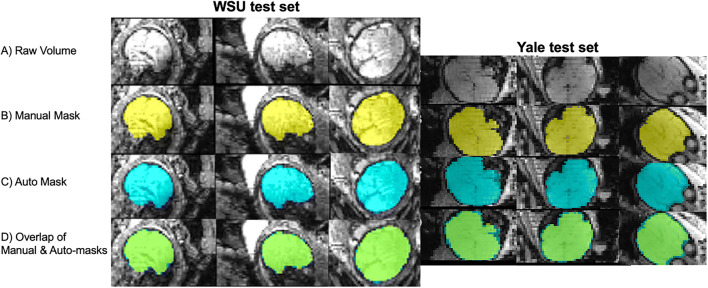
Comparison of manual and automated masks. (A) Raw volume; (B) Hand-drawn mask; (C) Auto mask; (D) Conjunction of hand drawn (yellow) and auto (blue) masks, overlap between hand and auto masks shown in green. *WSU data collected in Detroit, MI, at Wayne State University. Yale data collected in New Haven, CT at Yale University*
